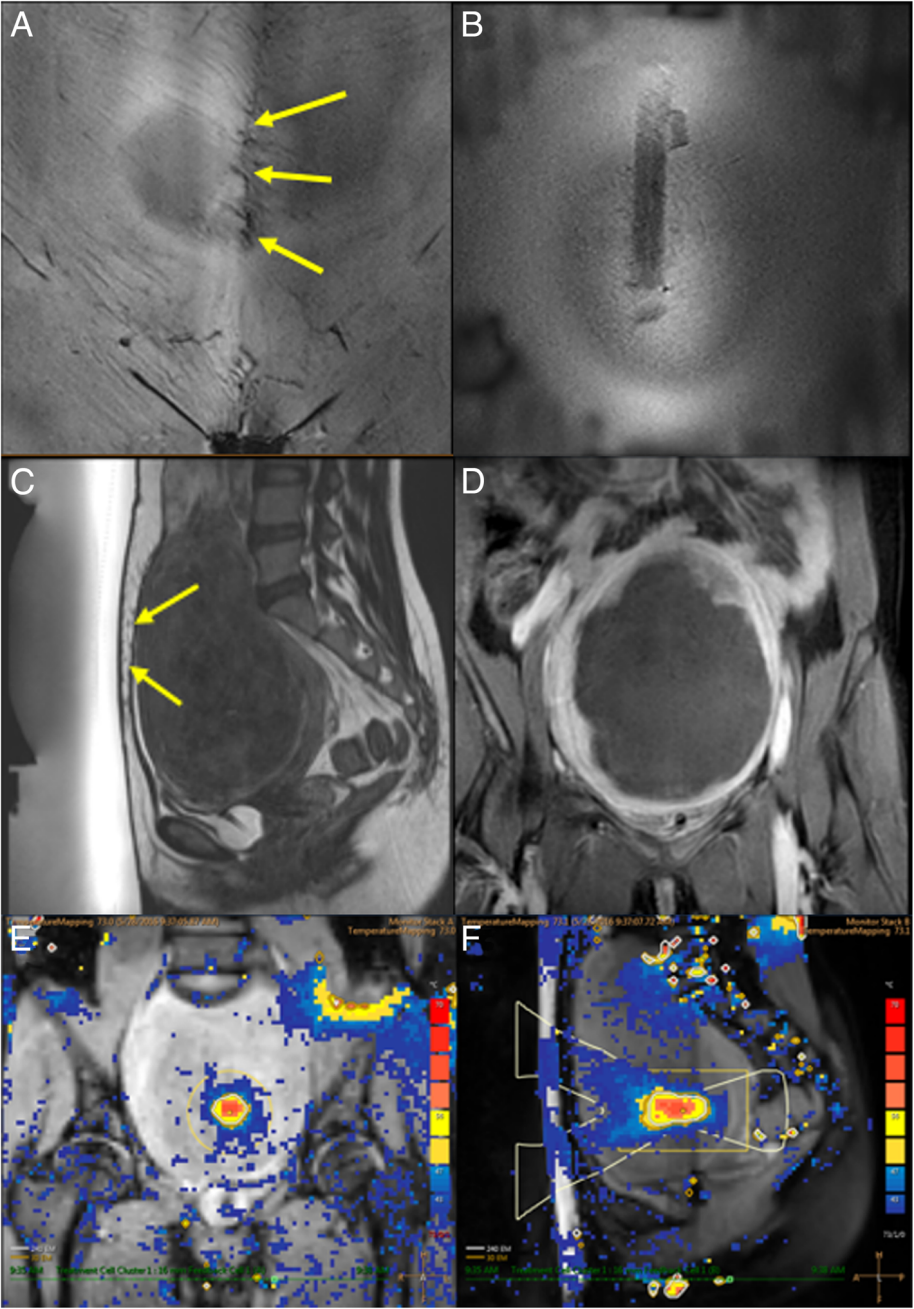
A 35-year-old woman with a 15.0-cm uterine fibroid, who had a 148-mm longitudinal abdominal scar, was treated with MRgHIFU ablation using a scar patch. a Scar imaging showing the orientation of the scar within the abdominal fat layer. Scar location identified with yellow arrows. b Scar imaging showing the air-containing scar patch at the patient’s skin. c Sagittal T2W planning MR image of uterine fibroid prior to high-intensity focused ultrasound treatment. Scar location identified with yellow arrows. d CE-T1W image acquired immediately after MRgHIFU treatment. Treatment success in terms of NPV ratio was 92%. e, f An example of multiplane MR thermometry acquired in both coronal and sagittal planes during one of the sonications
